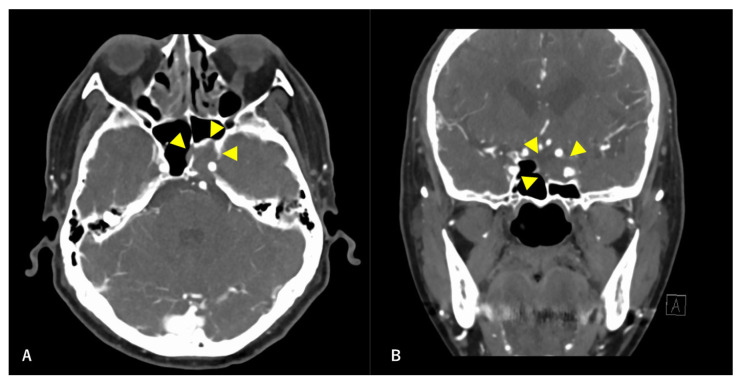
Preoperative contrast-enhanced computed tomography (CT) findings. Axial section (A) and coronal (B) images are shown. An isodense area with surrounding bone destruction is observed in the left sphenoid sinus, and no surrounding bone is observed around the internal carotid artery (ICA). Arrowheads indicate tumors.
